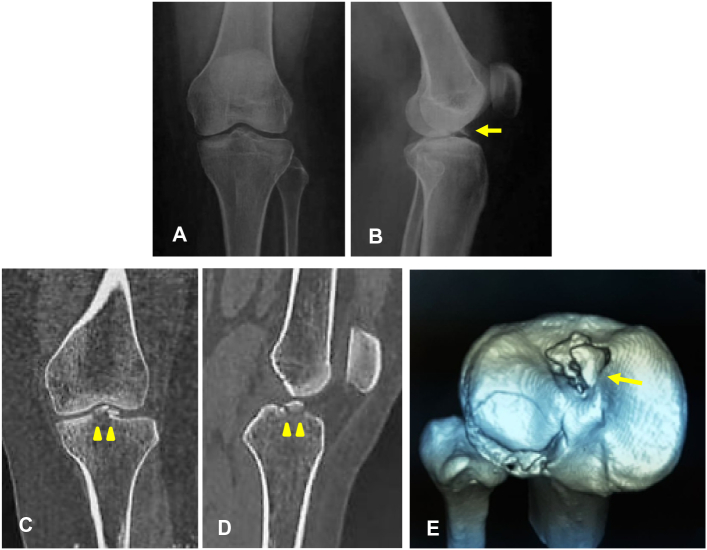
(A) The avulsion fracture of the ACL is not apparent on the frontal radiograph. (B) But lateral radiograph of the left knee confirms a displaced avulsion of the tibial eminence (yellow arrow). (C) Frontal section and, (D) Sagittal section of CT scan reveal displaced comminuted tibial eminence avulsion, (E) Three-dimensional CT image shows the avulsion fracture is comminuted involves entire of the ACL insertion (yellow arrow). (For interpretation of the references to colour in this figure legend, the reader is referred to the web version of this article.)
